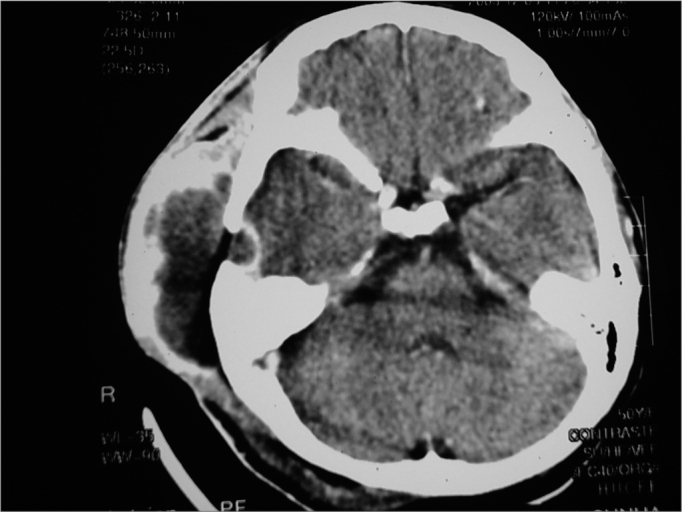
Mastoid CT scan, with a cystic soft tissue mass on the right temporal region, with peripheral contrast uptake, temporal lytic lesion on the right side with contrast uptake by the underlying dura matter.
